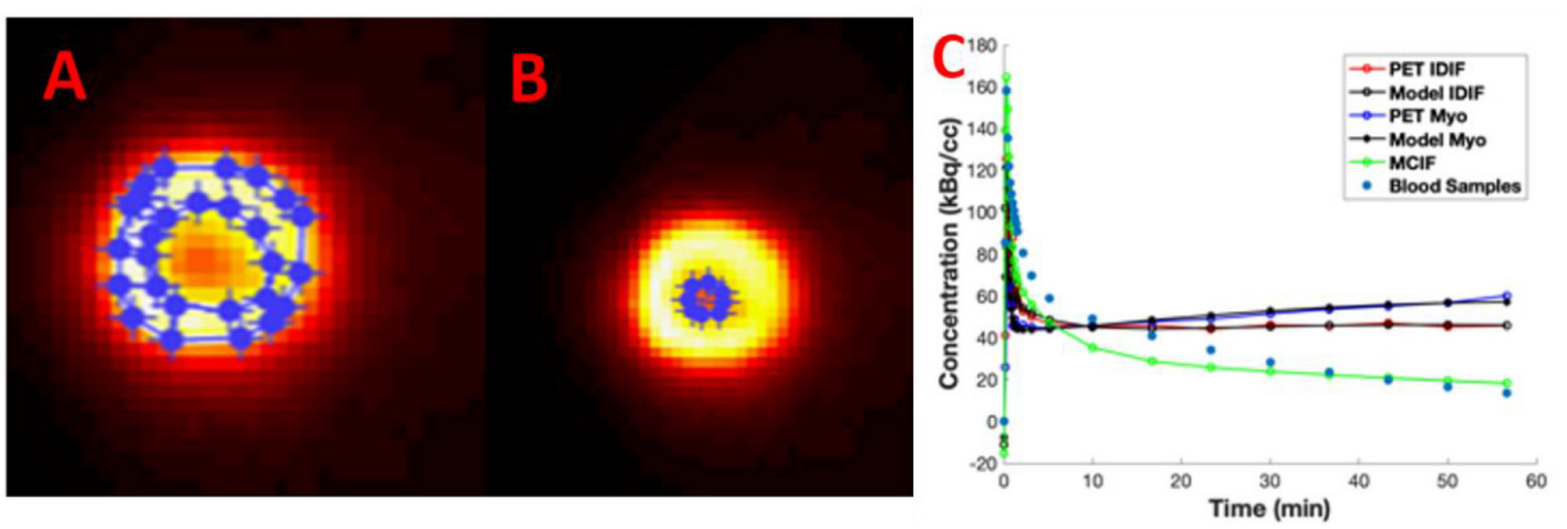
Model-corrected blood input function (MCIF). (A) Representative 2-[18F] fluoro-2-deoxy-D-glucose positron emission tomography (FDG PET) images with regions of interest drawn in the myocardium and (B) left ventricular blood in the last time frame. (C) Dual-output model (Model IDIF, Model Myo) fitted to image-derived blood (PET IDIF) and myocardium (PET Myo) time activity curves (TACs) to generate the MCIF. For comparison, blood samples derived from arterial blood sampling are shown.
